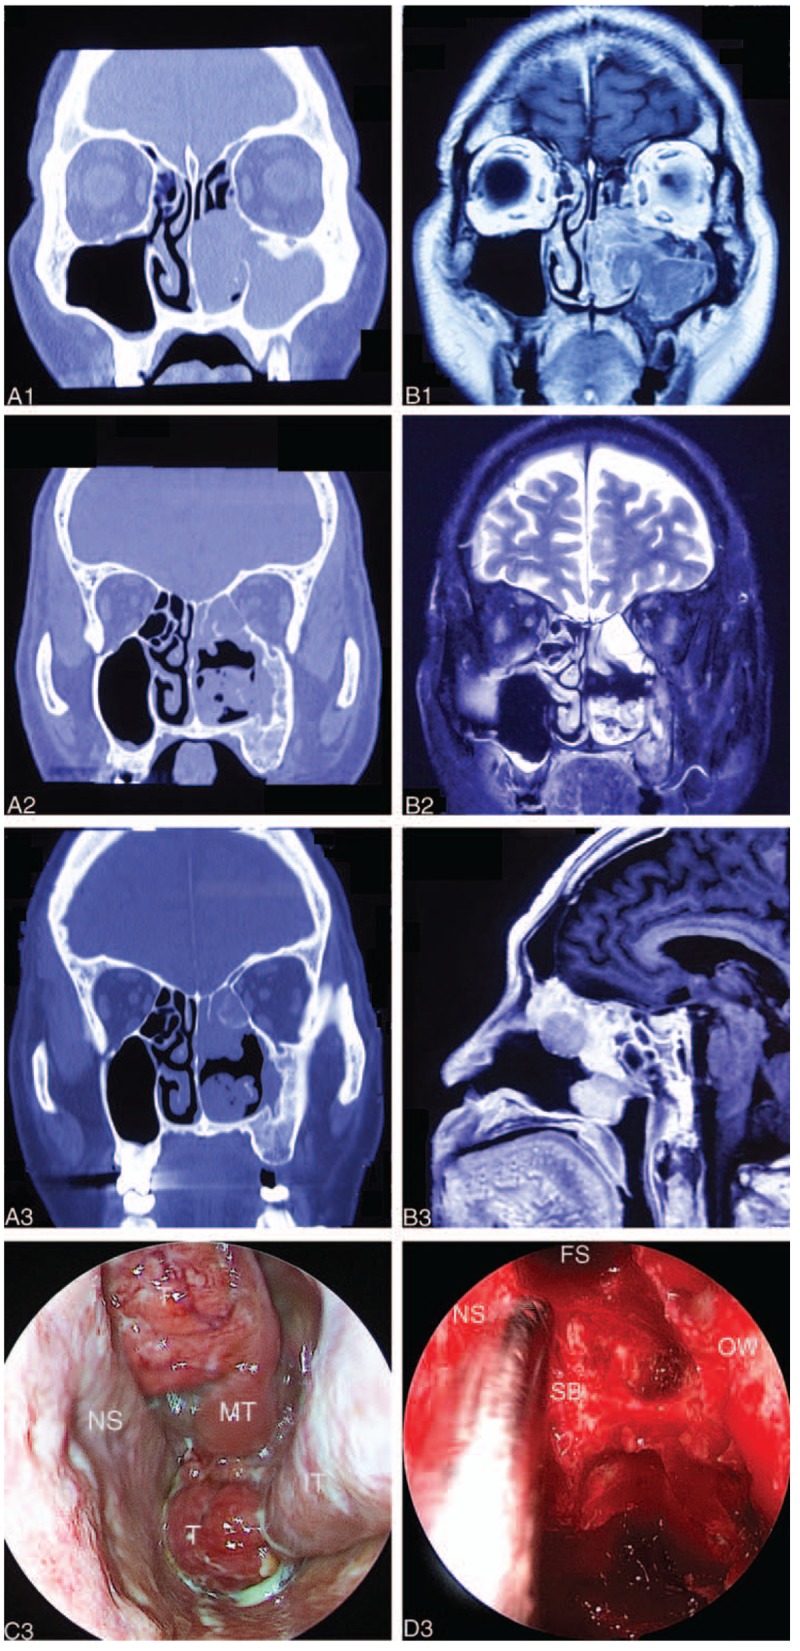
Imaging examination before the second (A1, B1), the third (A2, B2), and the fourth (A3, B3) surgery. The endoscopic view before and during the fourth surgery (C3, D3). (A1) Computed tomography (CT) scan showing osteitis around the left suborbital nerve canal (black arrow). (B1) T1-weighted, contrast-enhanced, fat-suppressed coronal MRI showing a massive left maxillary sinus neoplasm (black arrow). Note the striated appearance of the tumor. (A2) CT showing inflammatory response of the left maxillary bone (black arrow). (B2) MRI showing occupying lesion in the left nasal cavity, with absence of bony erosion into the orbit or skull base (black arrow). (A3, B3) CT and magnetic resonance images were similar to the last presurgery images. High inflammatory response of the left maxillary bone and 2 parts of the tumor were observed. Also, there was no bony erosion into the orbit or skull base. (C3) An endoscopic view before the fourth surgery. A neoplasm was located in the left nasal cavity, with a rough surface, easy bleeding, and more pus secretions on the surface of the tumor. (D3) Endoscopic intraoperative views showing anterior skull base exposure. FS = frontal sinus, IT = inferior turbinate, MT = middle turbinate, NS = nasal septum, OW = orbital wall, SB = skull base, T = tumor.
